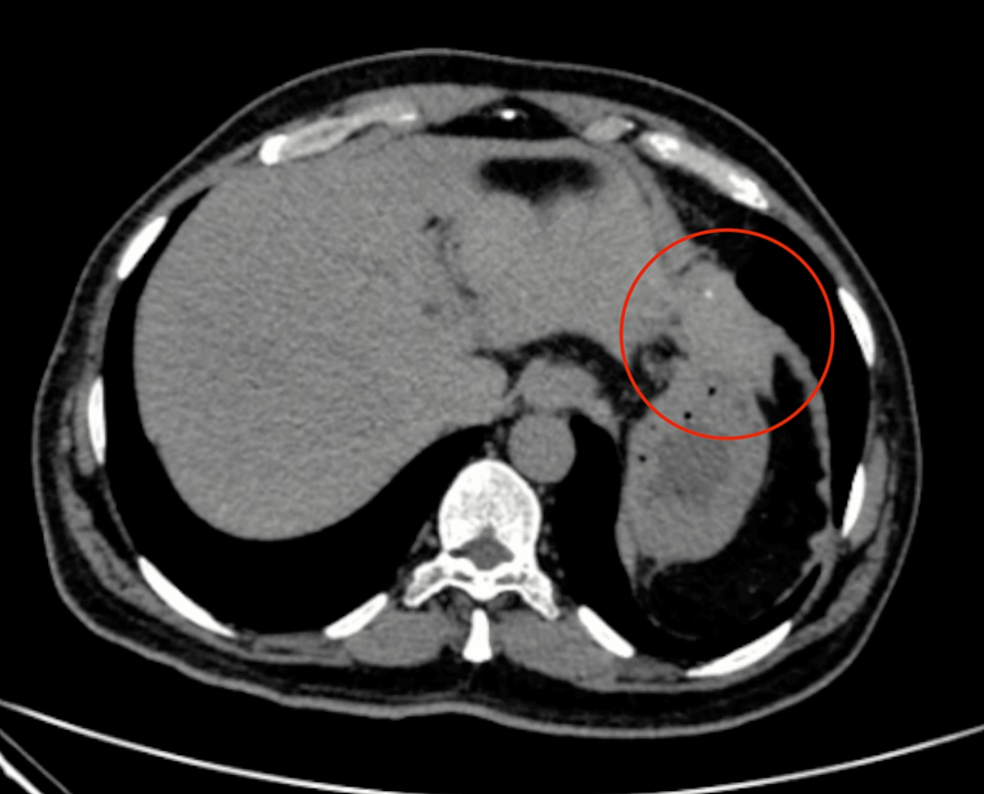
The suspected area on CT
